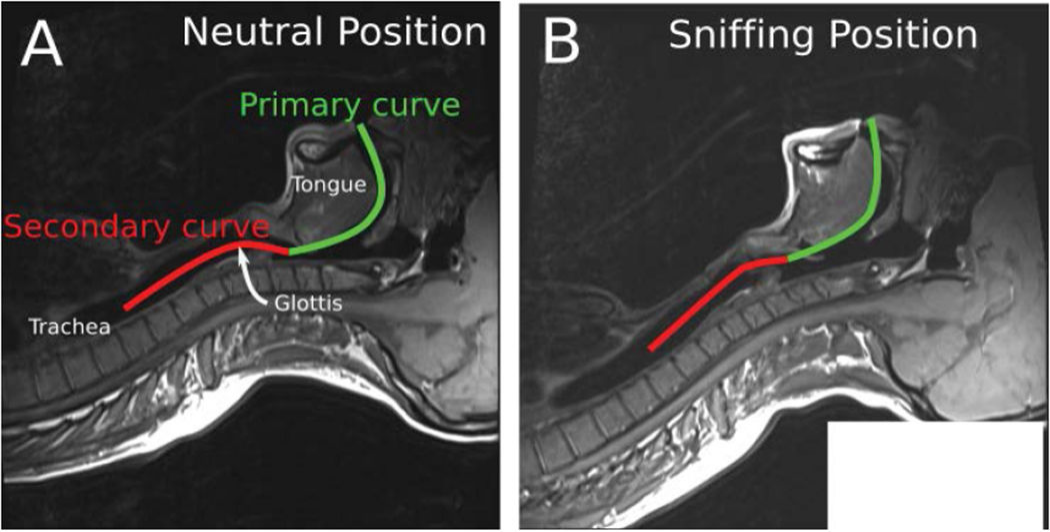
Alignment of the oral, laryngeal and pharyngeal axes with the intubator’s visual axis in the “Sniffing Position” as seen on an MRI scan [47]. Part of the figure was adopted from Greenland KB, et al. BJA. 2010;105(5):683-90. published by Elsevier with permission.
